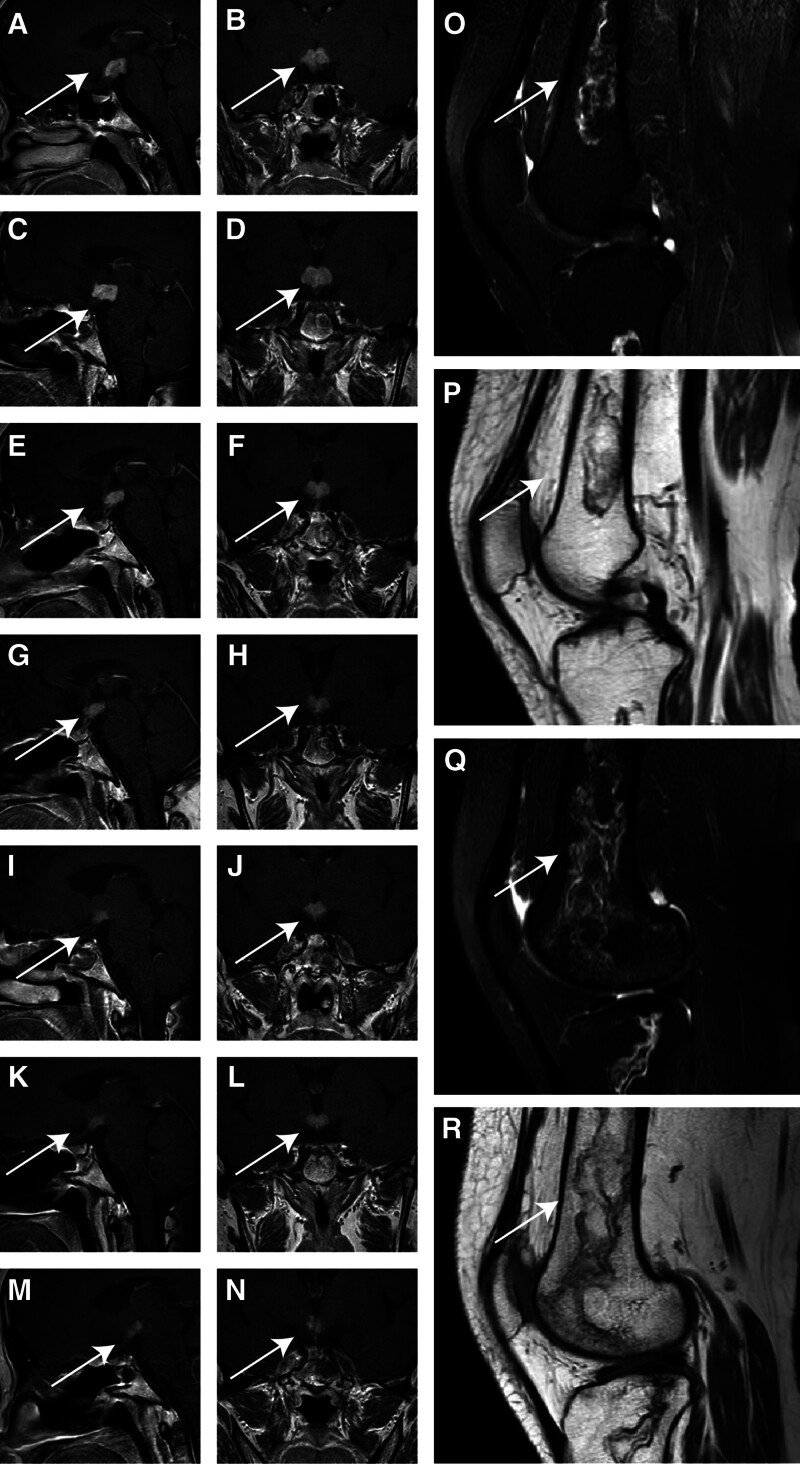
MRI of the serial pituitary and knee joints from 2023 to 2024. (A) to 1N show the patient’s pituitary sella region MRI scans. (A and B) are from February 2023, (C and D) are from January 2024, Figures (E and F) are from March 2024, (G and H) are from April 2024, (I and J) are from June 2024, (K and L) are from September 2024 and (M and N) are from June 2025. The arrows indicate the tumor mass. As shown in the images, the tumor mass gradually shrinks, with little change in size between June 2024 and June 2025. Figures 1O to 1R show the patient’s knee joint MRI scans. The arrows indicate the areas of bone infarction, with abnormal signals in the distal femurs and proximal tibias on both sides, suggesting femoral infarction.
